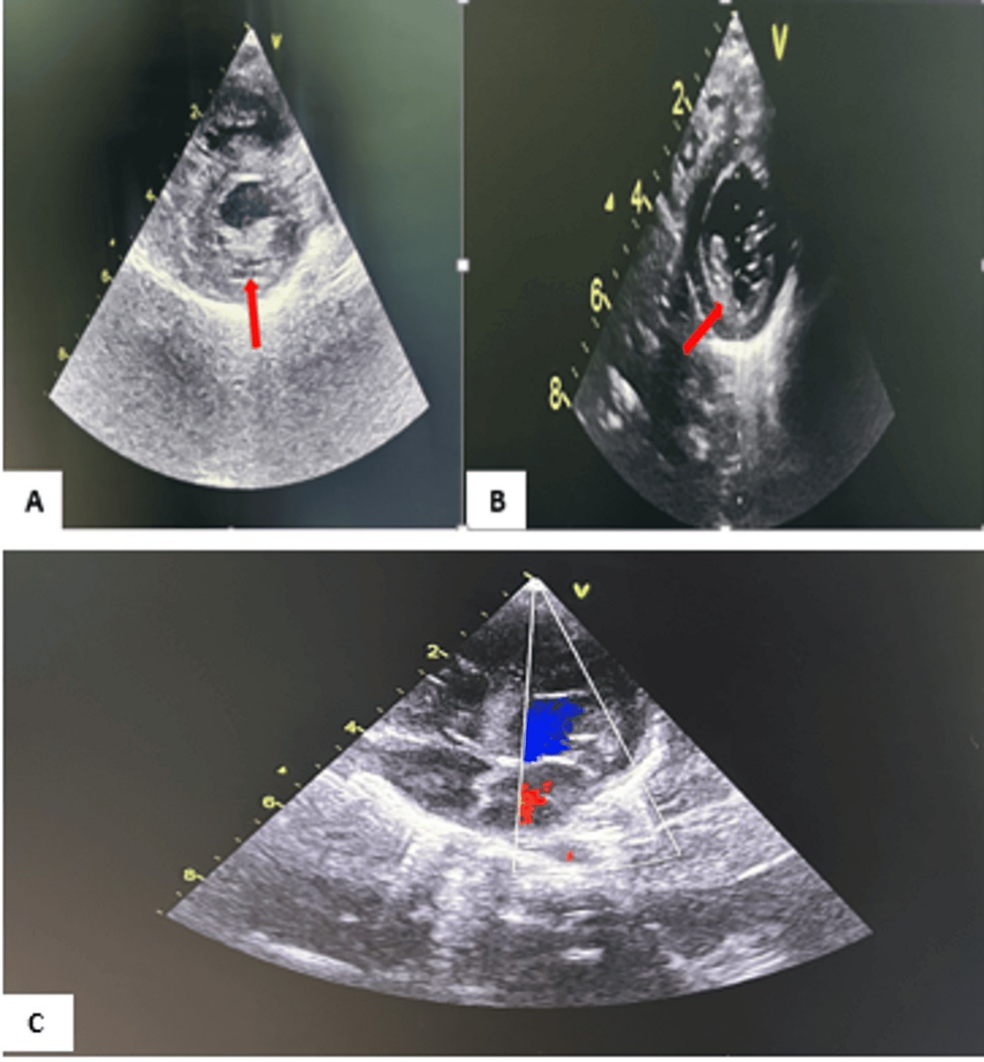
Echocardiographic image of the patient at admission. A: Short-axis parasternal section showing left ventricular hypertrophy with ejection fraction at 43%; B: short-axis parasternal section showing a 6 mm thickening of the posterior wall; C: four-chamber color Doppler imaging showing the overall cardiac morphology of our patient
